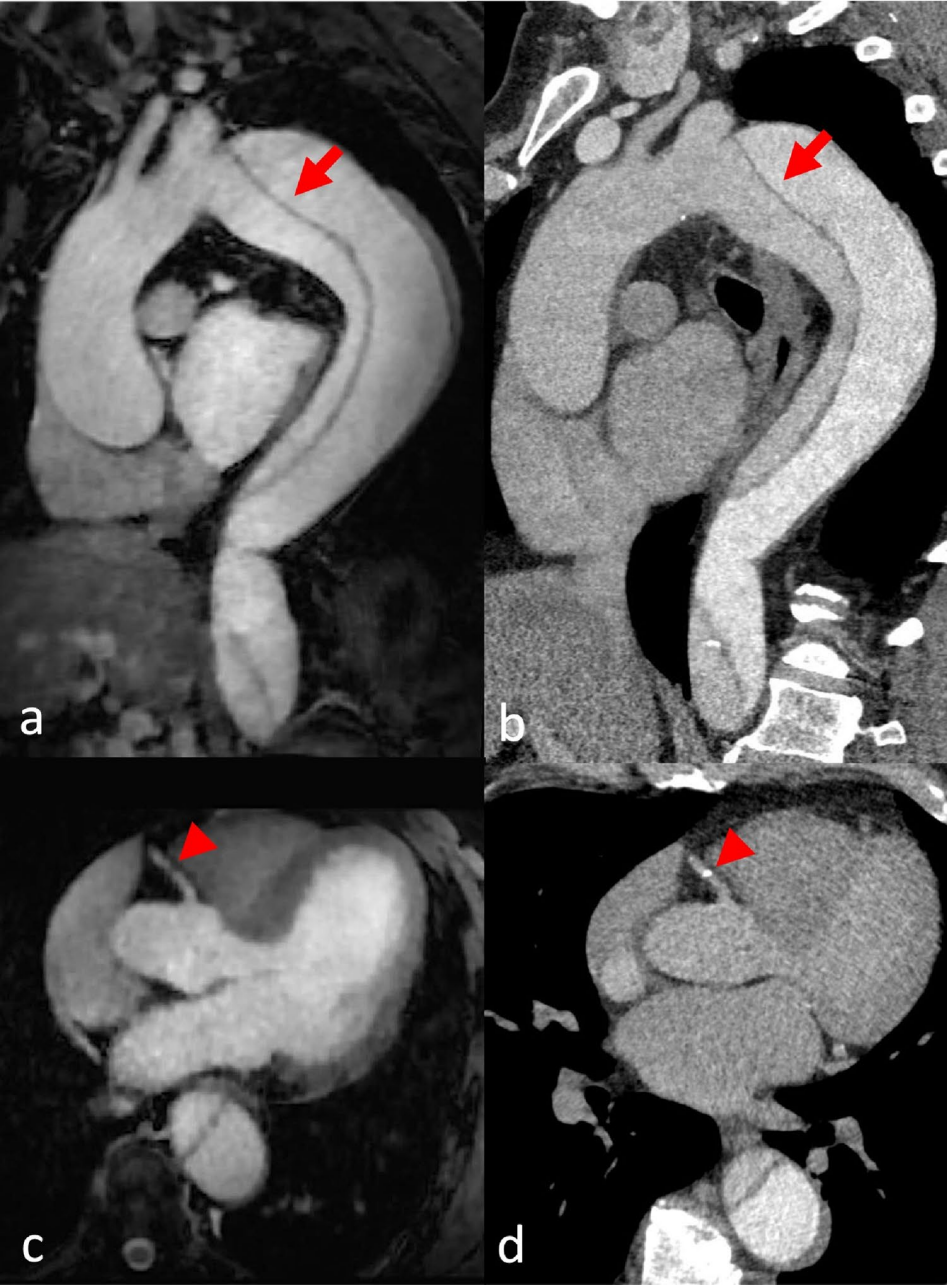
A patient with Stanford B aortic dissection was scheduled for follow-up imaging. Sagittal (a, b) and axial (c, d) reformations of MRA (a, c) and CTA (b, d). Good depiction in both modalities with unusual bolus timing in the CTA (a) due to a misplaced region of interest in the false lumen (arrow). Flow insensitivity renders both lumina hyperintense in MRA (b). A small calcification was found in the right coronary artery (arrowhead)
